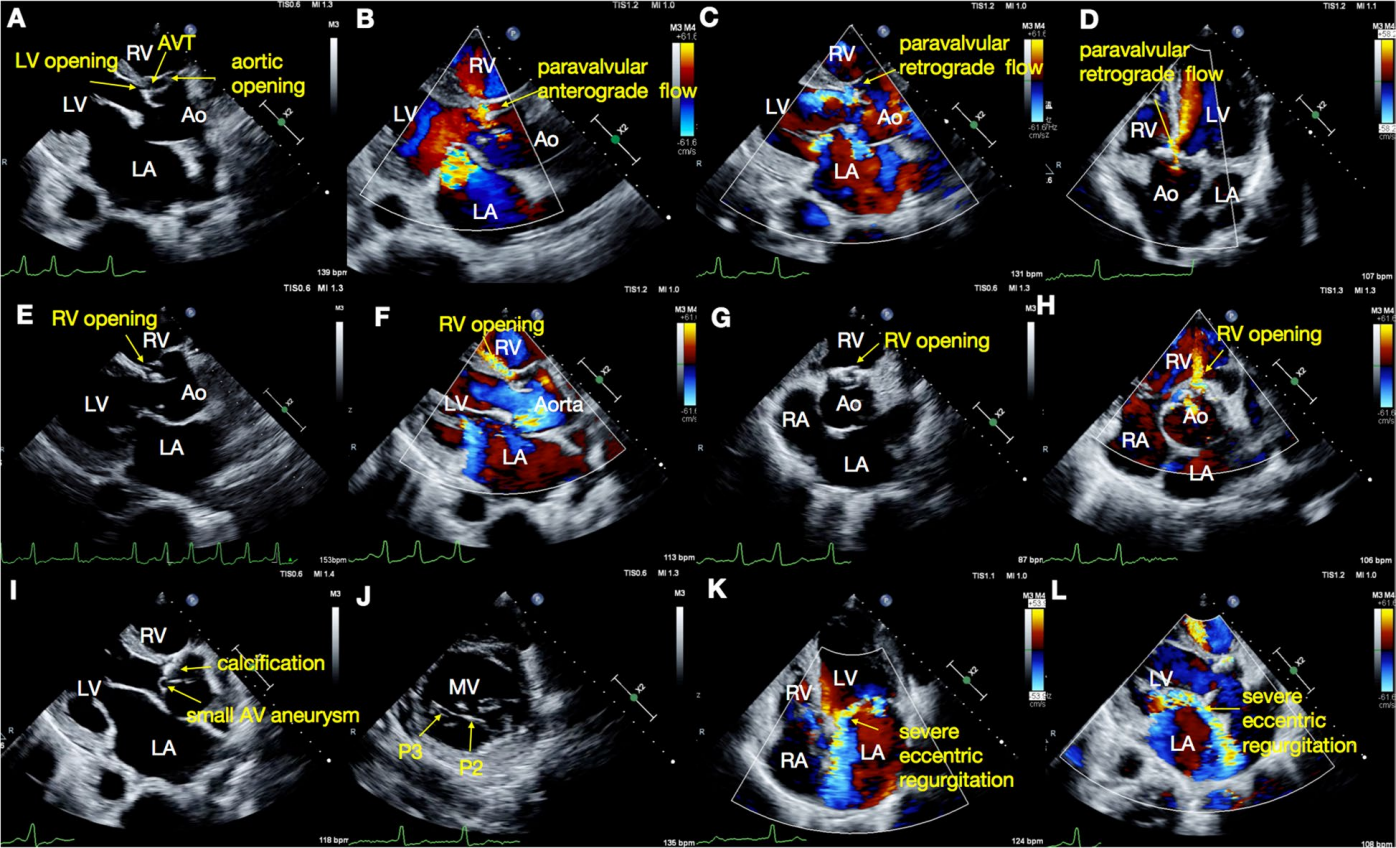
TTE images. A An AVT was noted, and its aortic and LV opening was observed in the parasternal long-axis view. B-C Color Doppler flow imaging (CDFI) revealed the anterograde systolic and retrograde diastolic flow between the aorta and the LV via the AVT in the parasternal long-axis view. D The retrograde diastolic flow via the AVT was also shown in the apical five-chamber view. E–F With a slight tilt of the probe, an RV opening was observed from the AVT in the modified parasternal long-axis view, and CDFI displayed a flow jet from the AVT to the RV. G-H The RV opening was also confirmed in the short-axis view. I The AV was calcified with a small aneurysm of the non-coronary cusp. J-L The MV chordal rupture of the P2 and P3 segments was observed in the posterior leaflet with severe eccentric regurgitation
